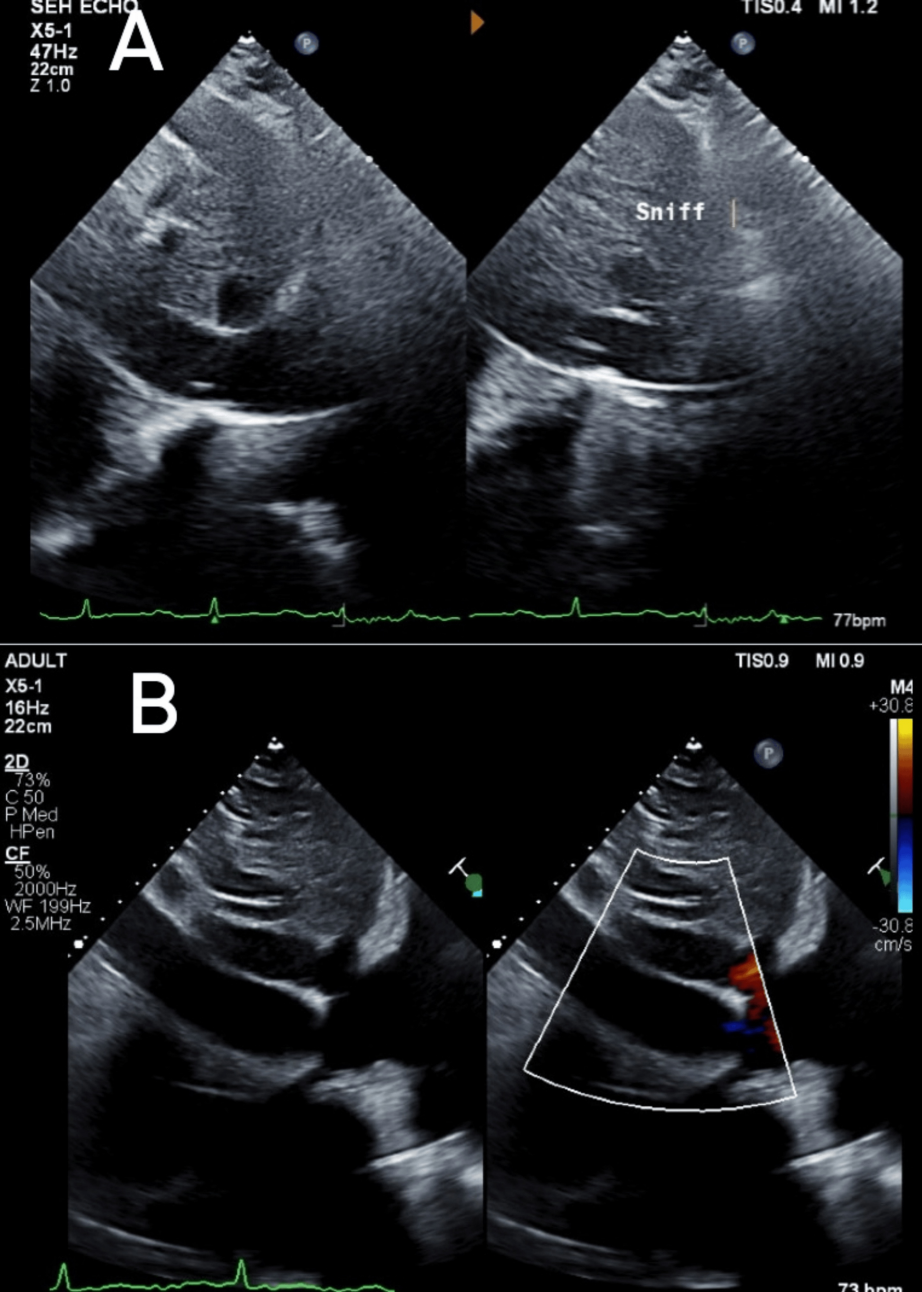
Transthoracic echocardiography in respirophasic cycle. Figure A shows reduced collapsibility of the inferior vena cava (IVC; 2.76 cm) on inspiration. Normally, it's collapsible in inspiration. Normal IVC diameter is 2.9 cm here. Figure B shows a plethoric IVC during inspiration.
